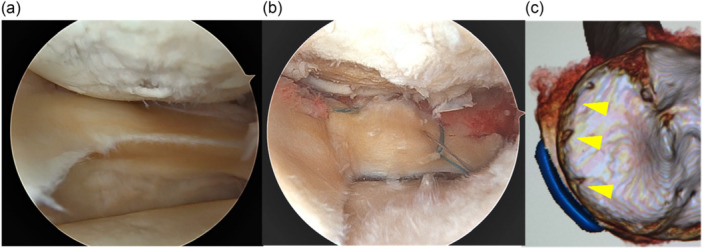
Intraoperative arthroscopy and postoperative three‐dimensional computed tomography. (a) Pre‐centralisation arthroscopic view. (b) Post‐centralisation arthroscopic view. (c) Three‐dimensional computed tomography showing anchor positions (yellow arrowheads).
